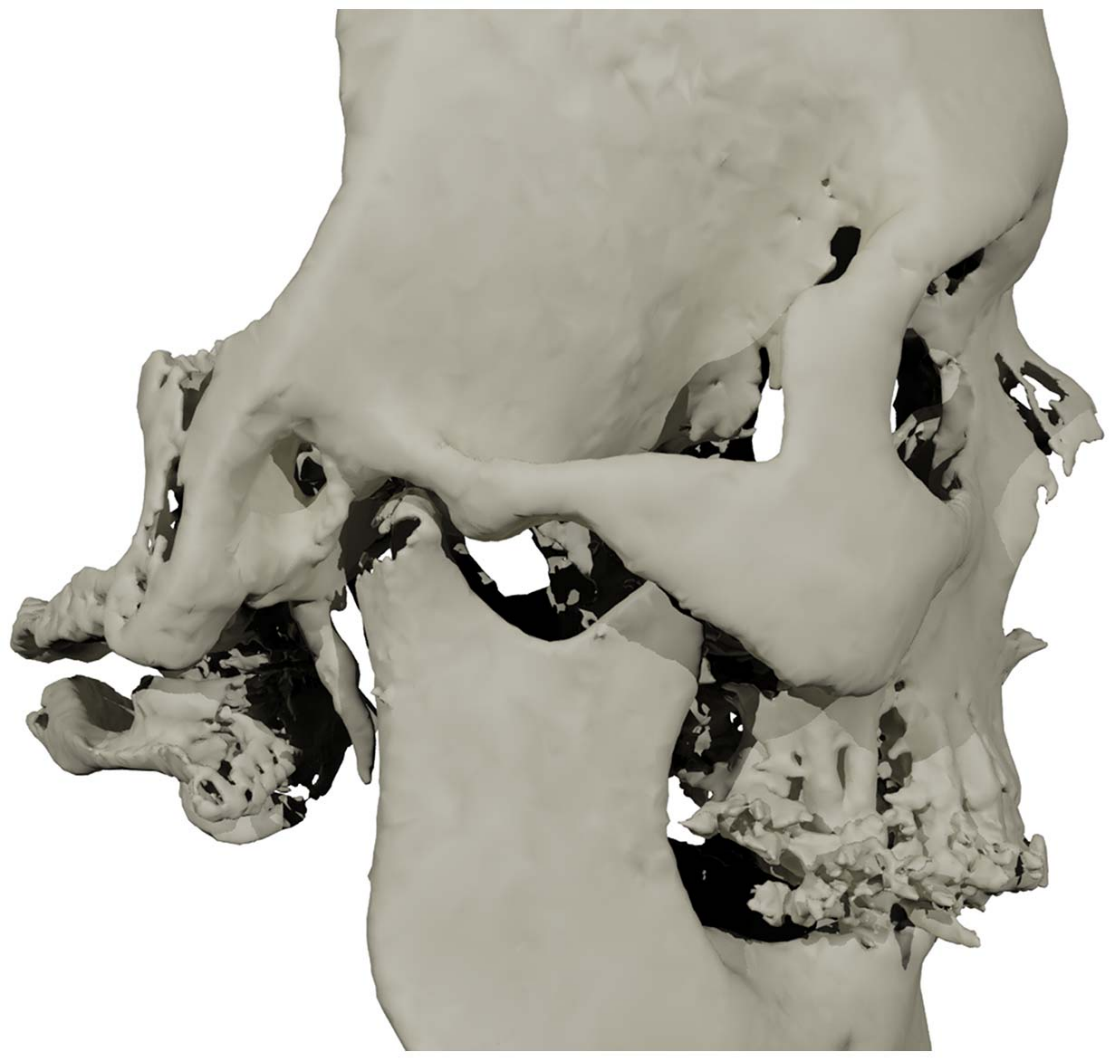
A 3D rendered virtual head model of a patient from Maxilim, reconstructed from the original CBCT data. The inaccurate reconstruction of the condyle is clearly visible. No measurement of the condylar shape or volume can be made due to the discontinuity of the condylar surface.
